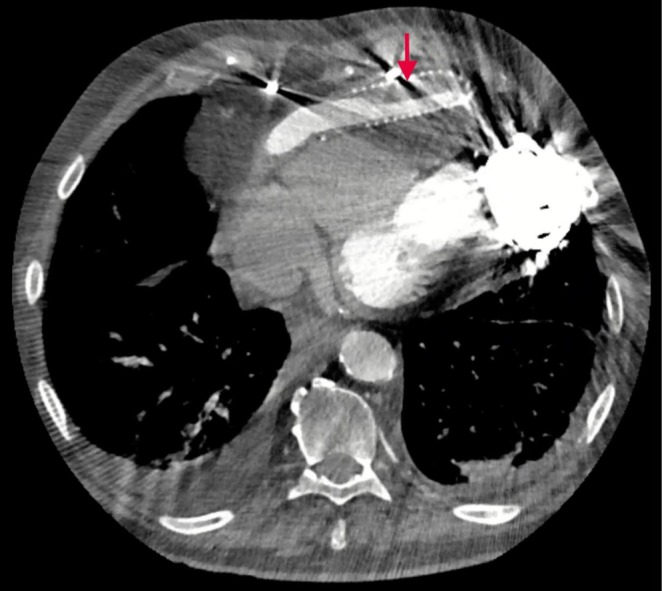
CT‐angiography image of a patient with eOGO (red arrow). *CT*, computed tomography; *eOGO*, external compression of the outflow graft causing obstruction. [Color figure can be viewed at wileyonlinelibrary.com]
